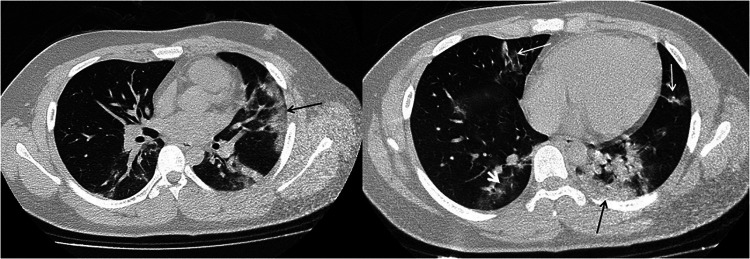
15-year-old boy infected with the original SARS-CoV-2. Initial unenhanced chest CT scans show multiple subpleural consolidations (black thin arrow) accompanied by ground glass opacities (white short arow) in lingual segment of left upper lobe and posterior basal segment of lower left lobe. Streaky opacities (white long arrow) are shown in right middle lobe and lingual segment of left upper lobe. CT findings were assigned CT severity score of 10.
